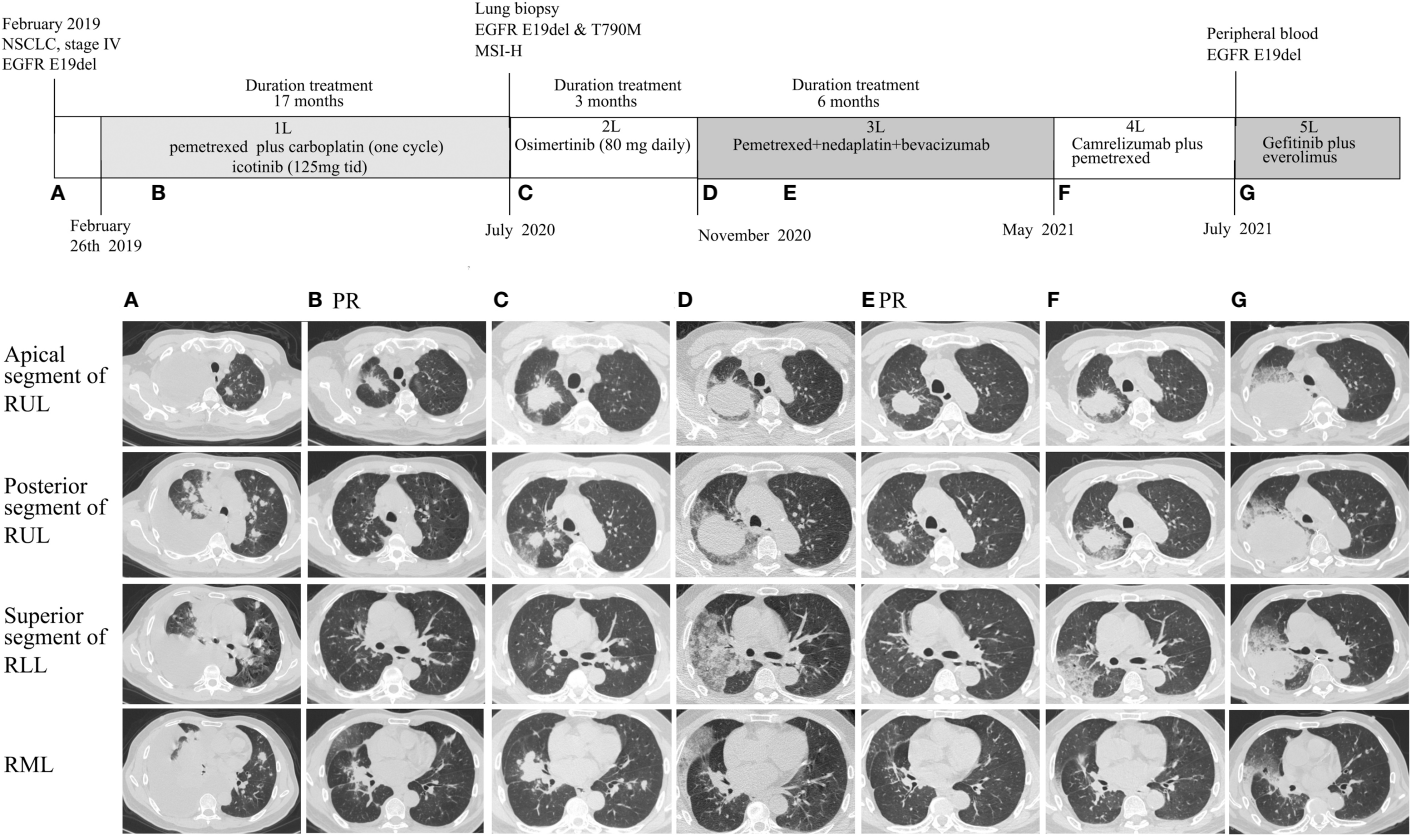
Radiographic evaluations of main lesions during the disease course. (A) Computed tomography (CT) scan at diagnosis. (B) CT scan showed lesions shrank after 1 month of first-line icotinib; (C) CT scan after resistance to icotinib. (D) CT scan after 3 months of osimertinib treatment; (E) CT scan before the second cycle of nedaplatin–pemetrexed–bevacizumab showing partial response; (F) CT after 6 months of third-line therapy showing disease progression; (G) CT scan after 2 months of immunotherapy. RUL, right upper lobe; RLL, right lower lobe; RML, right middle lobe; PR, partial response.
